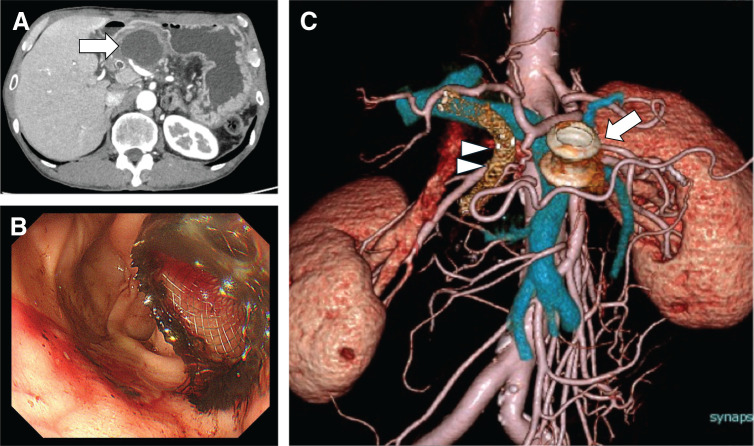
Endoscopic management and preoperative anatomical assessment. (A) The pancreatic pseudocyst progressed to infectious pancreatic necrosis during chemotherapy. (B) Endoscopic ultrasound-guided transgastric drainage using a lumen-apposing metal stent. (C) Reconstructed CT image demonstrates vascular anatomy and the location of the biliary and lumen-apposing metal stents.
